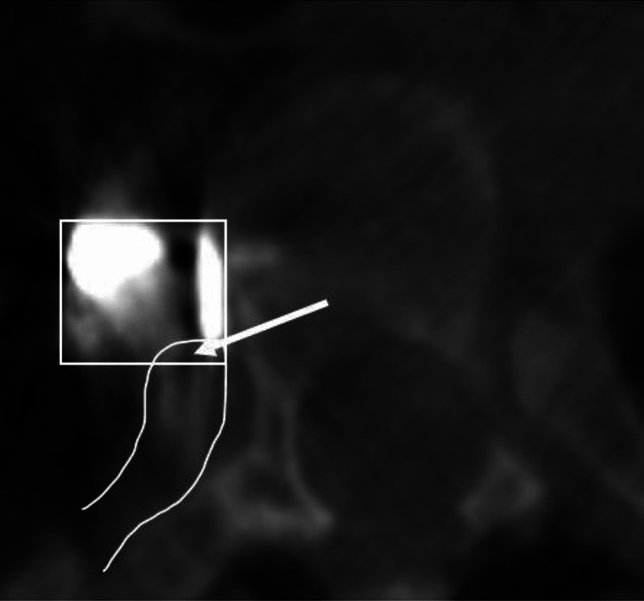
Axial CT image showing documentation of the head of a VBT screw (square) at its insertion point impinging on an outlined thoracic rib head
